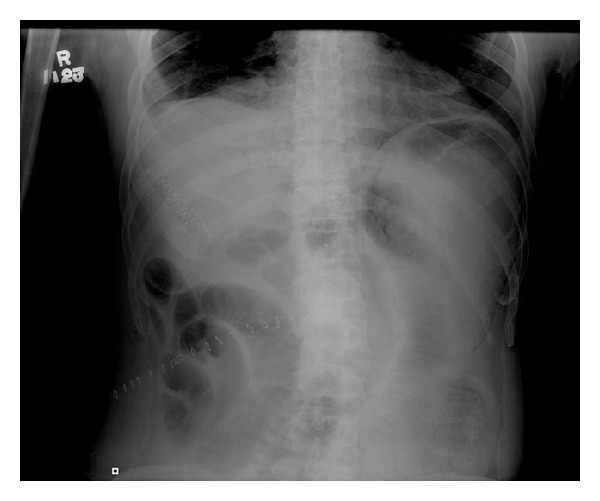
Abdominal radiograph on postoperative day 4: lucency in the stomach and small bowel ileus.
